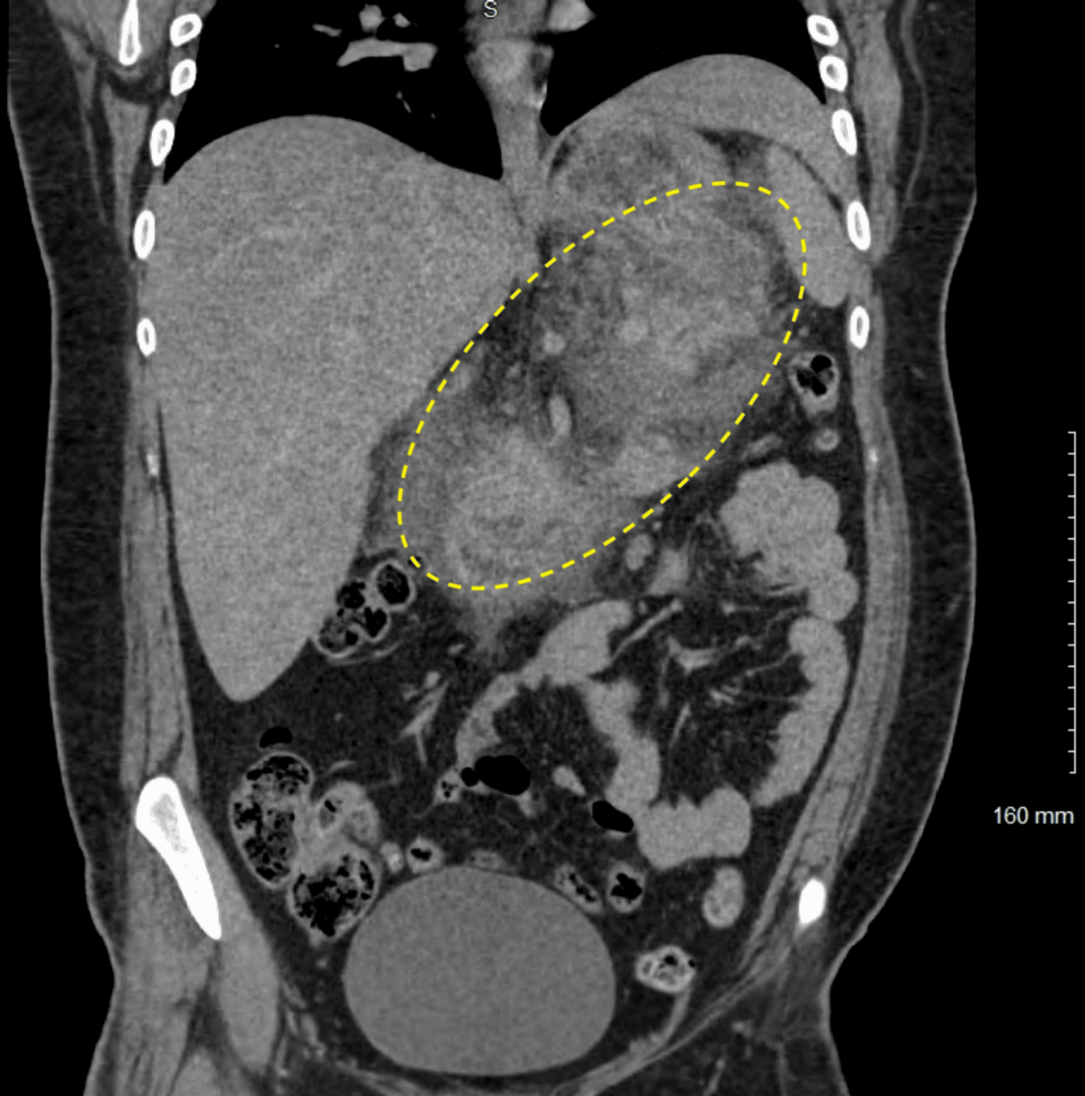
Coronal section of computed tomography reveals pancreatitis and peri-pancreatic inflammation
